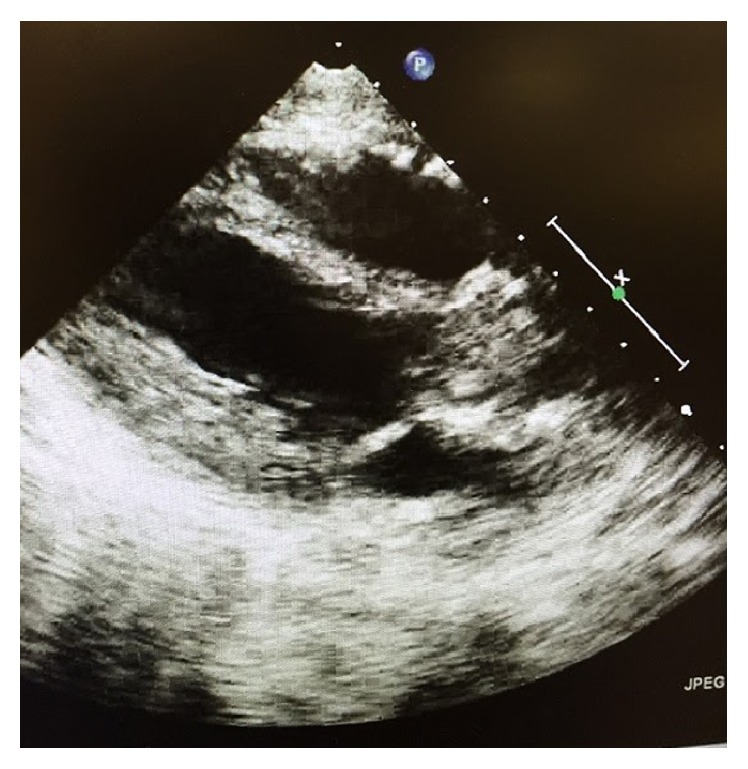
Repeat echocardiogram showing larger left ventricle with markedly reduced function, as well as mitigation of previously overloaded right ventricular size and function.
